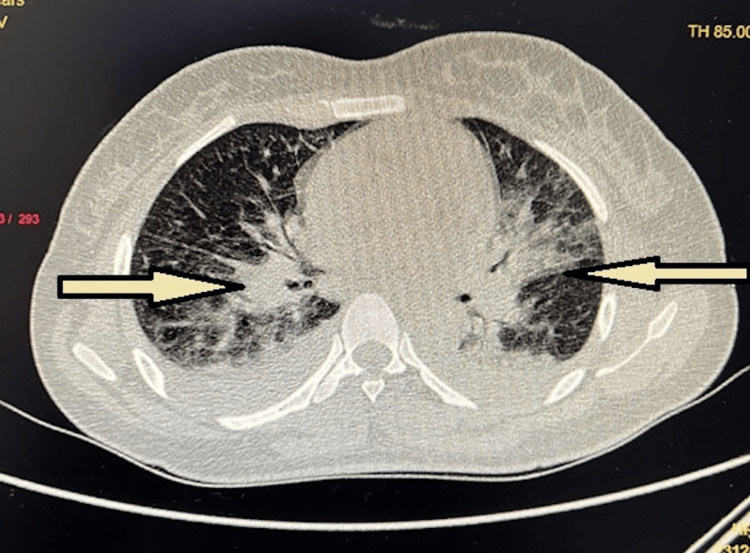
The high-resolution computed tomography (HRCT) scan showed several patches of background consolidation and patchy ground glass opacities (arrows). The image is original and produced by the authors
